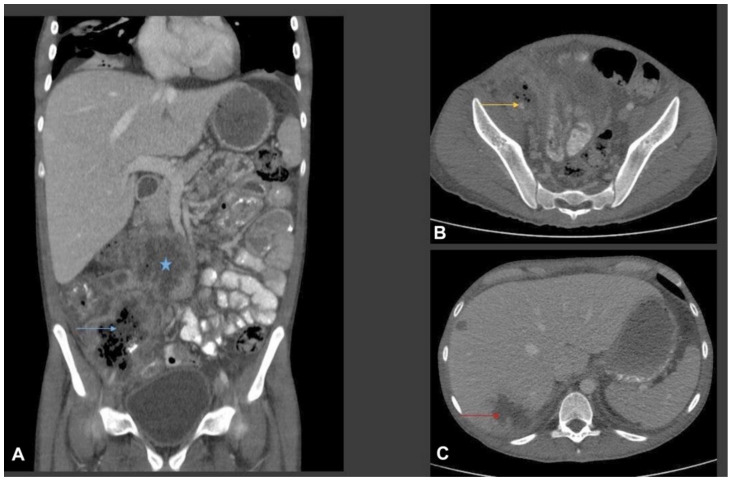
Multiplanar CT scan of abdomen with oral and intravenous contrast. (A) Coronal CT image shows a multispatial ill-defined low-attenuation ring enhancing collection in the root of the mesentery (star) and in the right iliac fossa. The right iliac fossa collection shows thick irregular rim enhancement with central bubbly air lucency (blue arrow). Bowel loops are adherent to the collections. There is marked inflammatory fat stranding noted in the right iliac fossa and right side of the pelvic cavity. Small volume ascites. (B) Axial CT image from the right iliac fossa and pelvis shows marked phlegmonous soft tissue lesion with fat stranding. The phlegmonous inflammatory mass is encasing the right external iliac artery which is small and irregular in calibre (yellow arrow). (C) Axial images from the liver show multifocal ill-defined low-attenuation liver lesions of variable size (two of them shown in this image). The largest was seen in the subcapsular liver in the segment VII and shows surround oedema, suggestive of liver abscess (red arrow).
